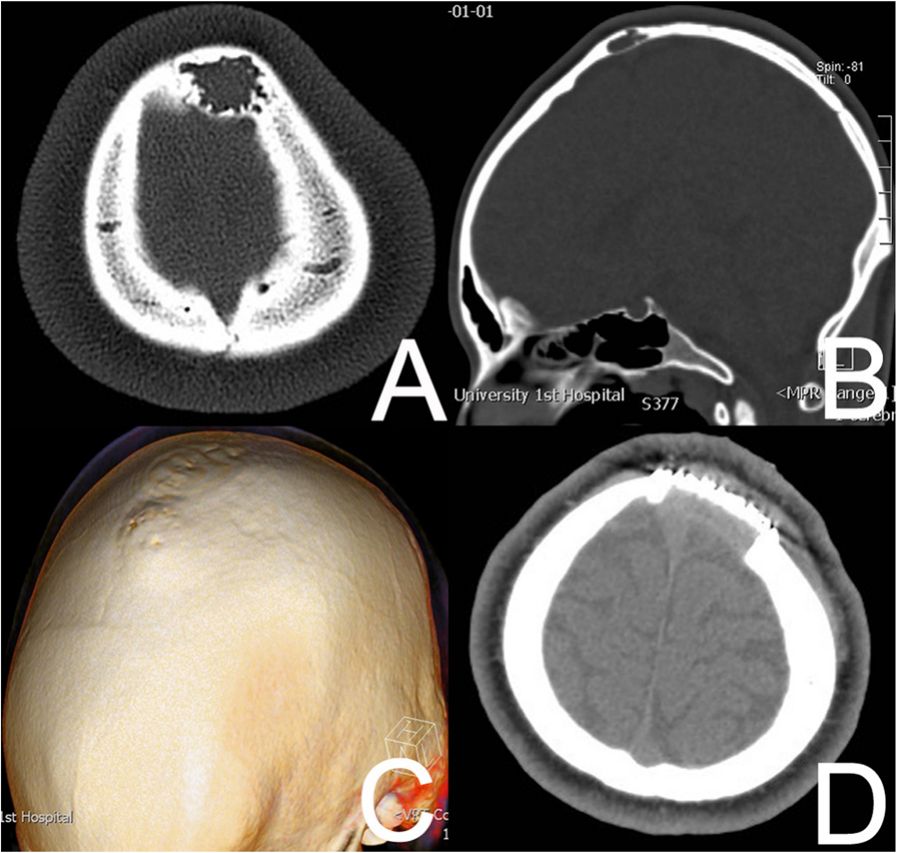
Preoperative CT images of bone-window revealed a low density mass, irregular shape, internal and external bony plates eroded. (A) On axial CT scan. (B) On sagittal CT scan. (C) A 3D reconstructed image by CT showed external bony plates to be jacked up. (D) Postoperative CT scan of the axial showing no tumor recurrence 12 months after operation.
